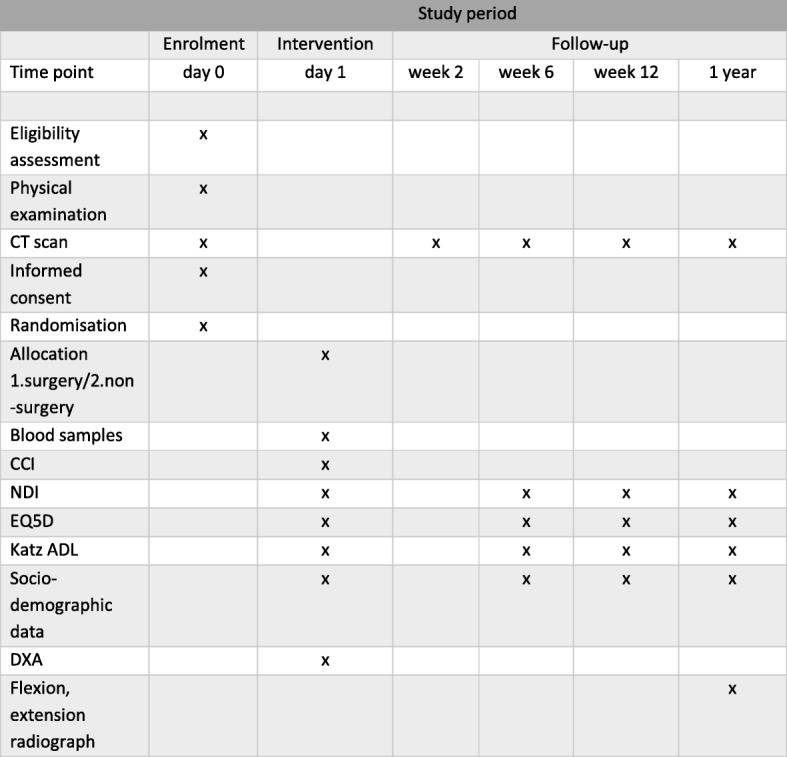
Participant timeline. *CT* computed tomography, *CCI* charlson comorbidity index, *EQ-5D* euroQol, *NDI* neck disability index, katz *ADL* activities in daily life according to katz, *DXA* double x-ray absorptiometry
